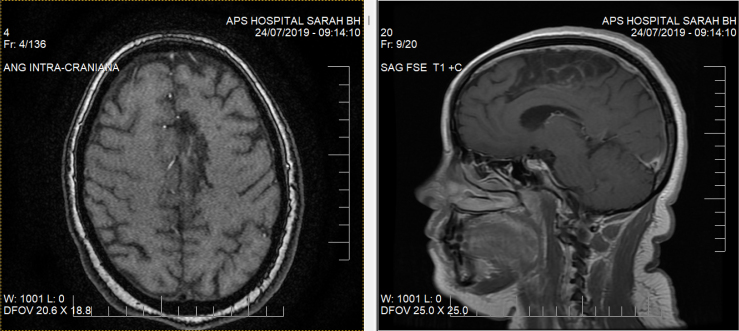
Magnetic resonance imaging showing encephalomalacia area in branches of the left anterior cerebral territory, covering most of supplementary motor area (Brodmann area 6).
